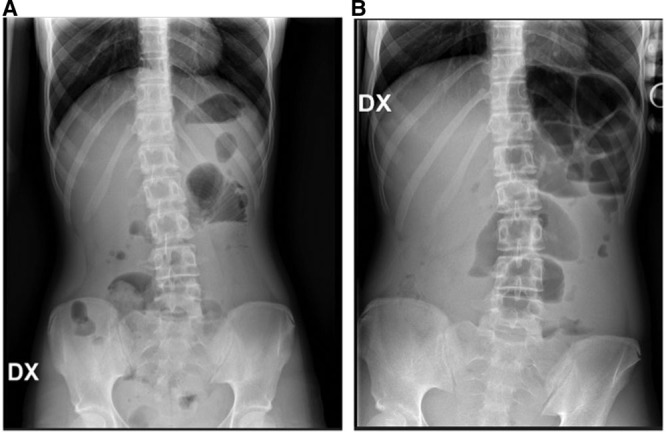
A) Plain abdominal x-rays showing bowel obstruction: air-fluid levels at the left upper quadrant with colonic gas distension. B) Worsening abdominal occlusion demonstrated by increased air-fluid levels.
